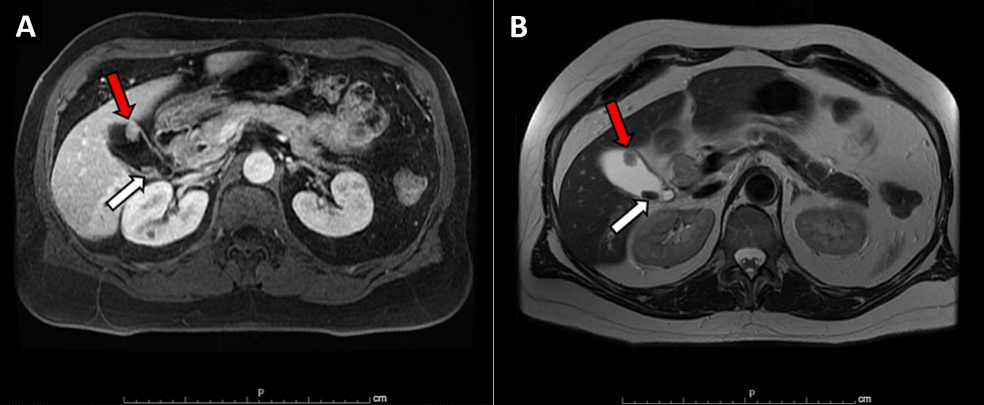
MRI showing gallbladder adenoma and intraluminal gallstone (A) MRI venous phase post-contrast shows a 1.6 x 1.2 cm enhancing mass in the gallbladder (red arrow) and intraluminal gallstone (white arrow). (B) T2 half-Fourier acquisition single-shot turbo spin-echo (HASTE) shows the same findings as above.
